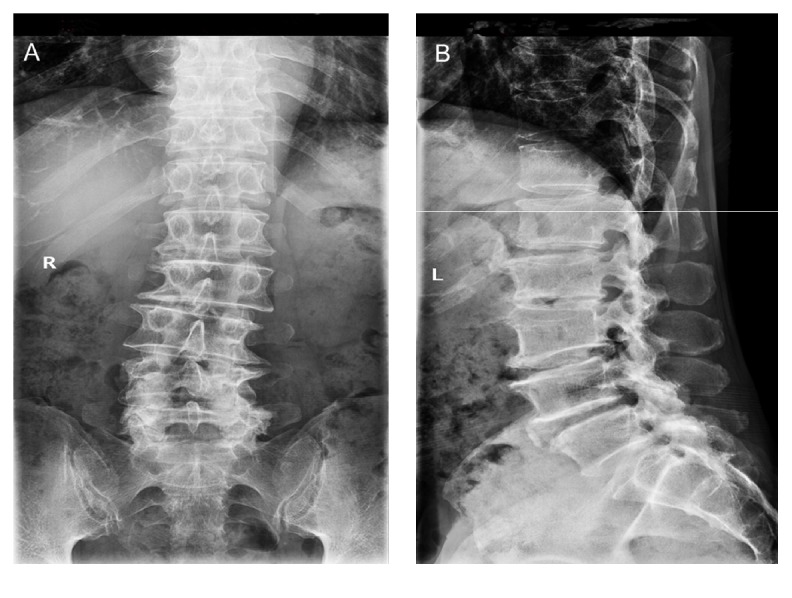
The preoperative anteroposterior (A) and left lateral (B) X-ray images of the patient, showing lumbar scoliosis, lumbar degeneration, and vertebral instability.
